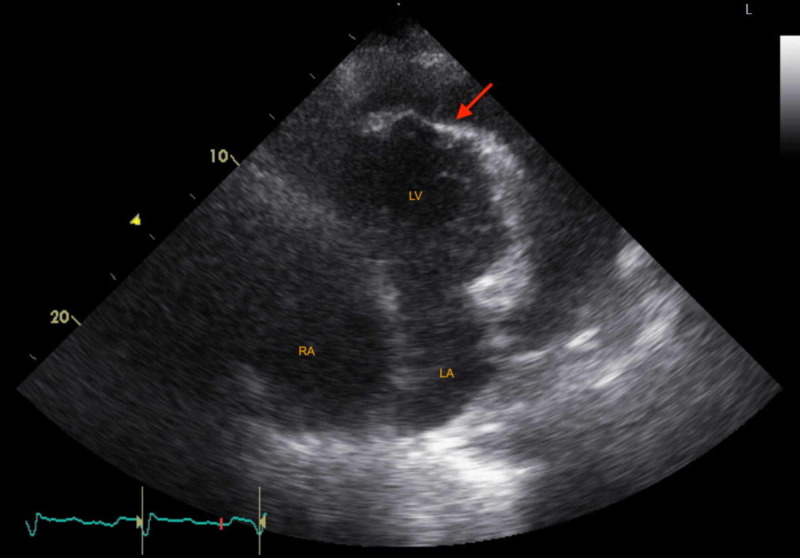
Transthoracic echocardiogram with LV collapse Apical-four chamber view showing large pericardial effusion with diastolic LV apical collapse (arrow) LA: left atrium; LV: left ventricle; RA: right atrium
